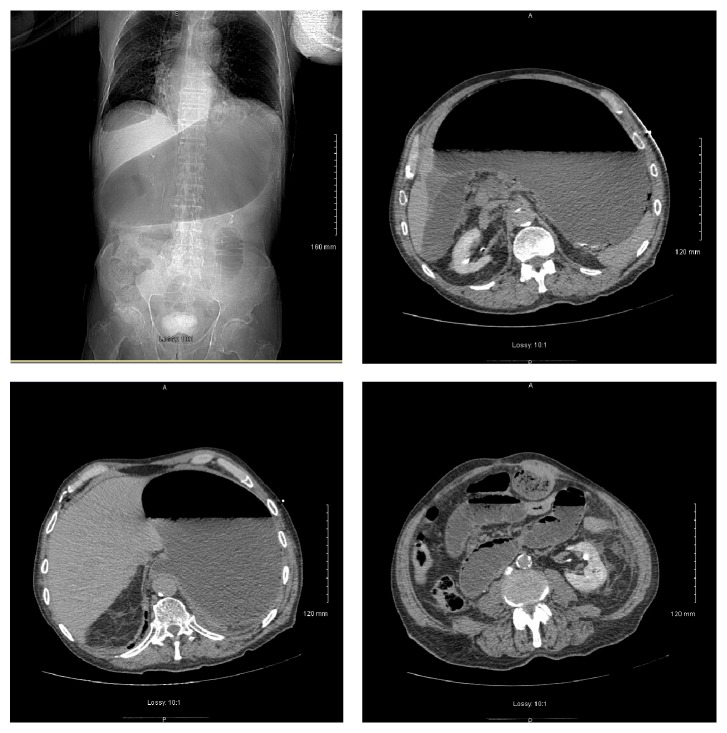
CT scan of the abdomen showing small bowel obstruction, with transition point in the jejunum with a markedly dilated stomach containing foci of air. Also a dilated gall bladder with gallstones and normal-appearing pancreas is noted.
